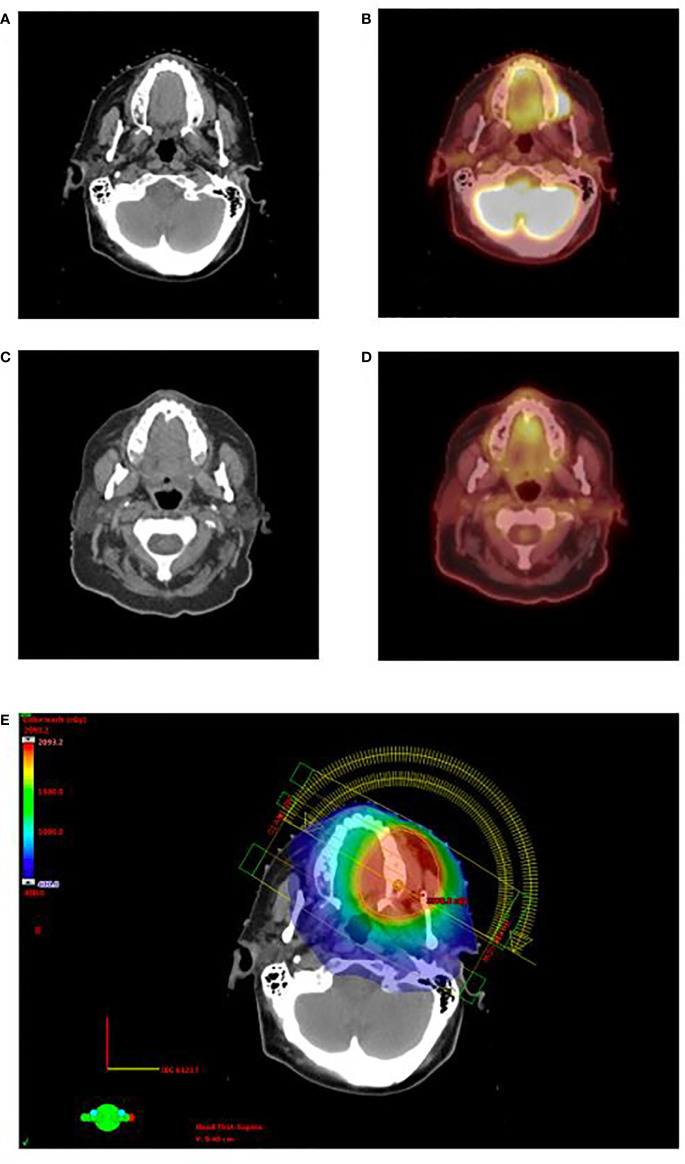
Clinical appearance of gingival MS in the left superior alveolar ridge for patient 1 on pretreatment CT (A) and PET/CT (B). The patient demonstrated complete response on CT (C) and PET/CT (D) 3 months after the completion of RT. Rapid arc IMRT treatment plan is shown in (E). CT, computed tomography; PET/CT, positron emission tomography/computed tomography; RT, radiotherapy; IMRT, Intensity modulated radiation therapy.
